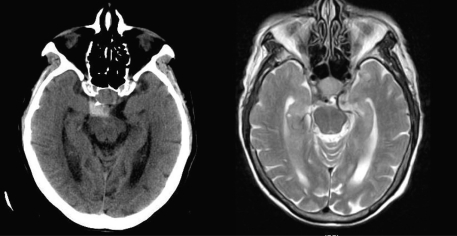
Axial views of head CT (left) and MRI T2 (right) showing the pituitary hemorrhage (CT) and the pituitary macroadenoma (MRI).
